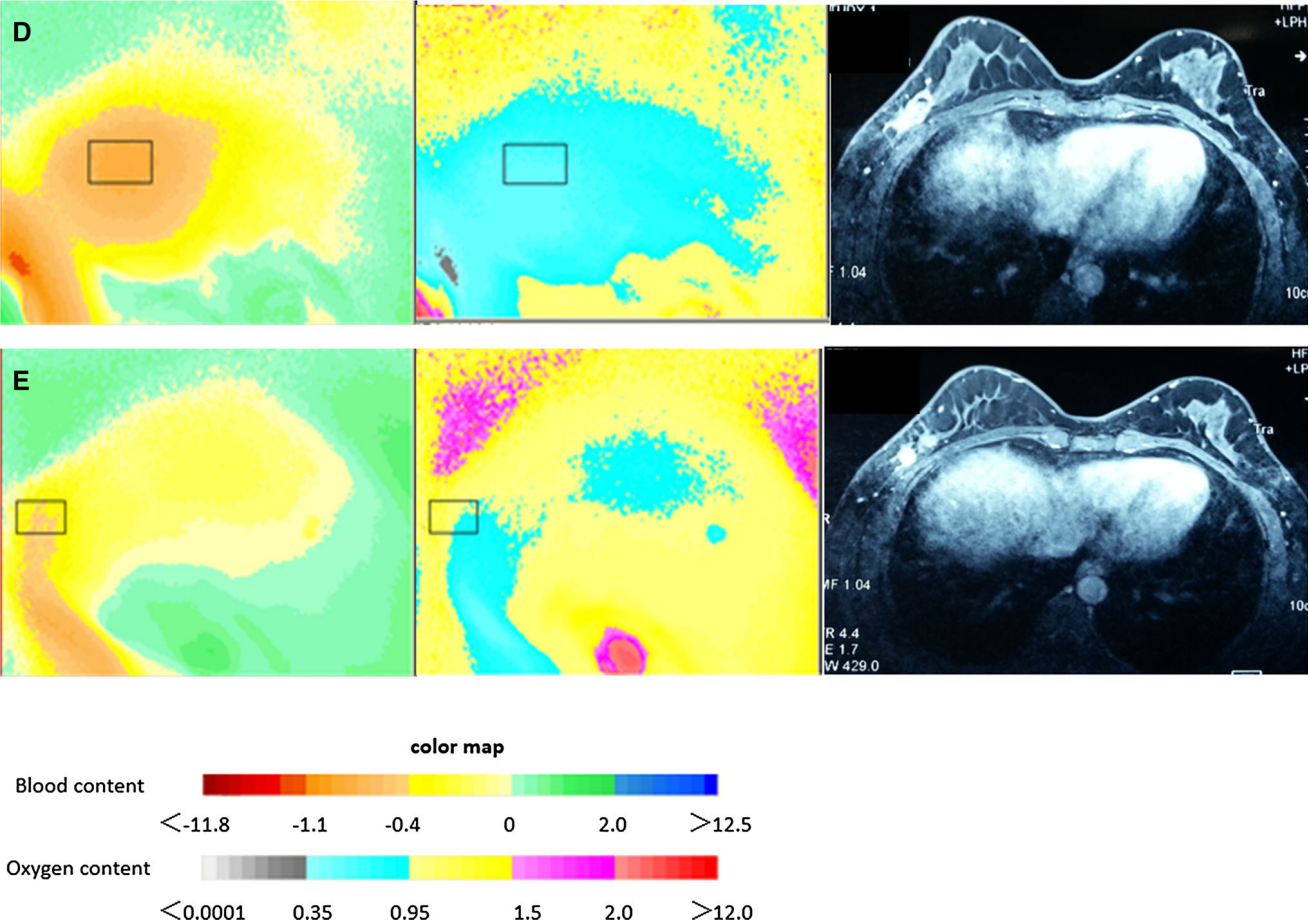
The corresponding blood-oxygen images and MRI of a typical breast cancer responder who completed five NAC treatment cycle before surgery. The figures from A to E present the change of the malignant tumor in blood-oxygen images and MRI. Left, blood value image; median, oxygen value image; right, MRI image. The colormap shows the parameter of blood-oxygen content of tumor lesion. In blood value image, *Red area* presents high blood content; in oxygen value image, *blue area* means low oxygen content. The image of malignant tumor always presents high blood and low oxygen. It was presented that the blood content decreased and the oxygen content increased, which was a typical responder’s change. As a control, the tumor size in MRI became more and more small
